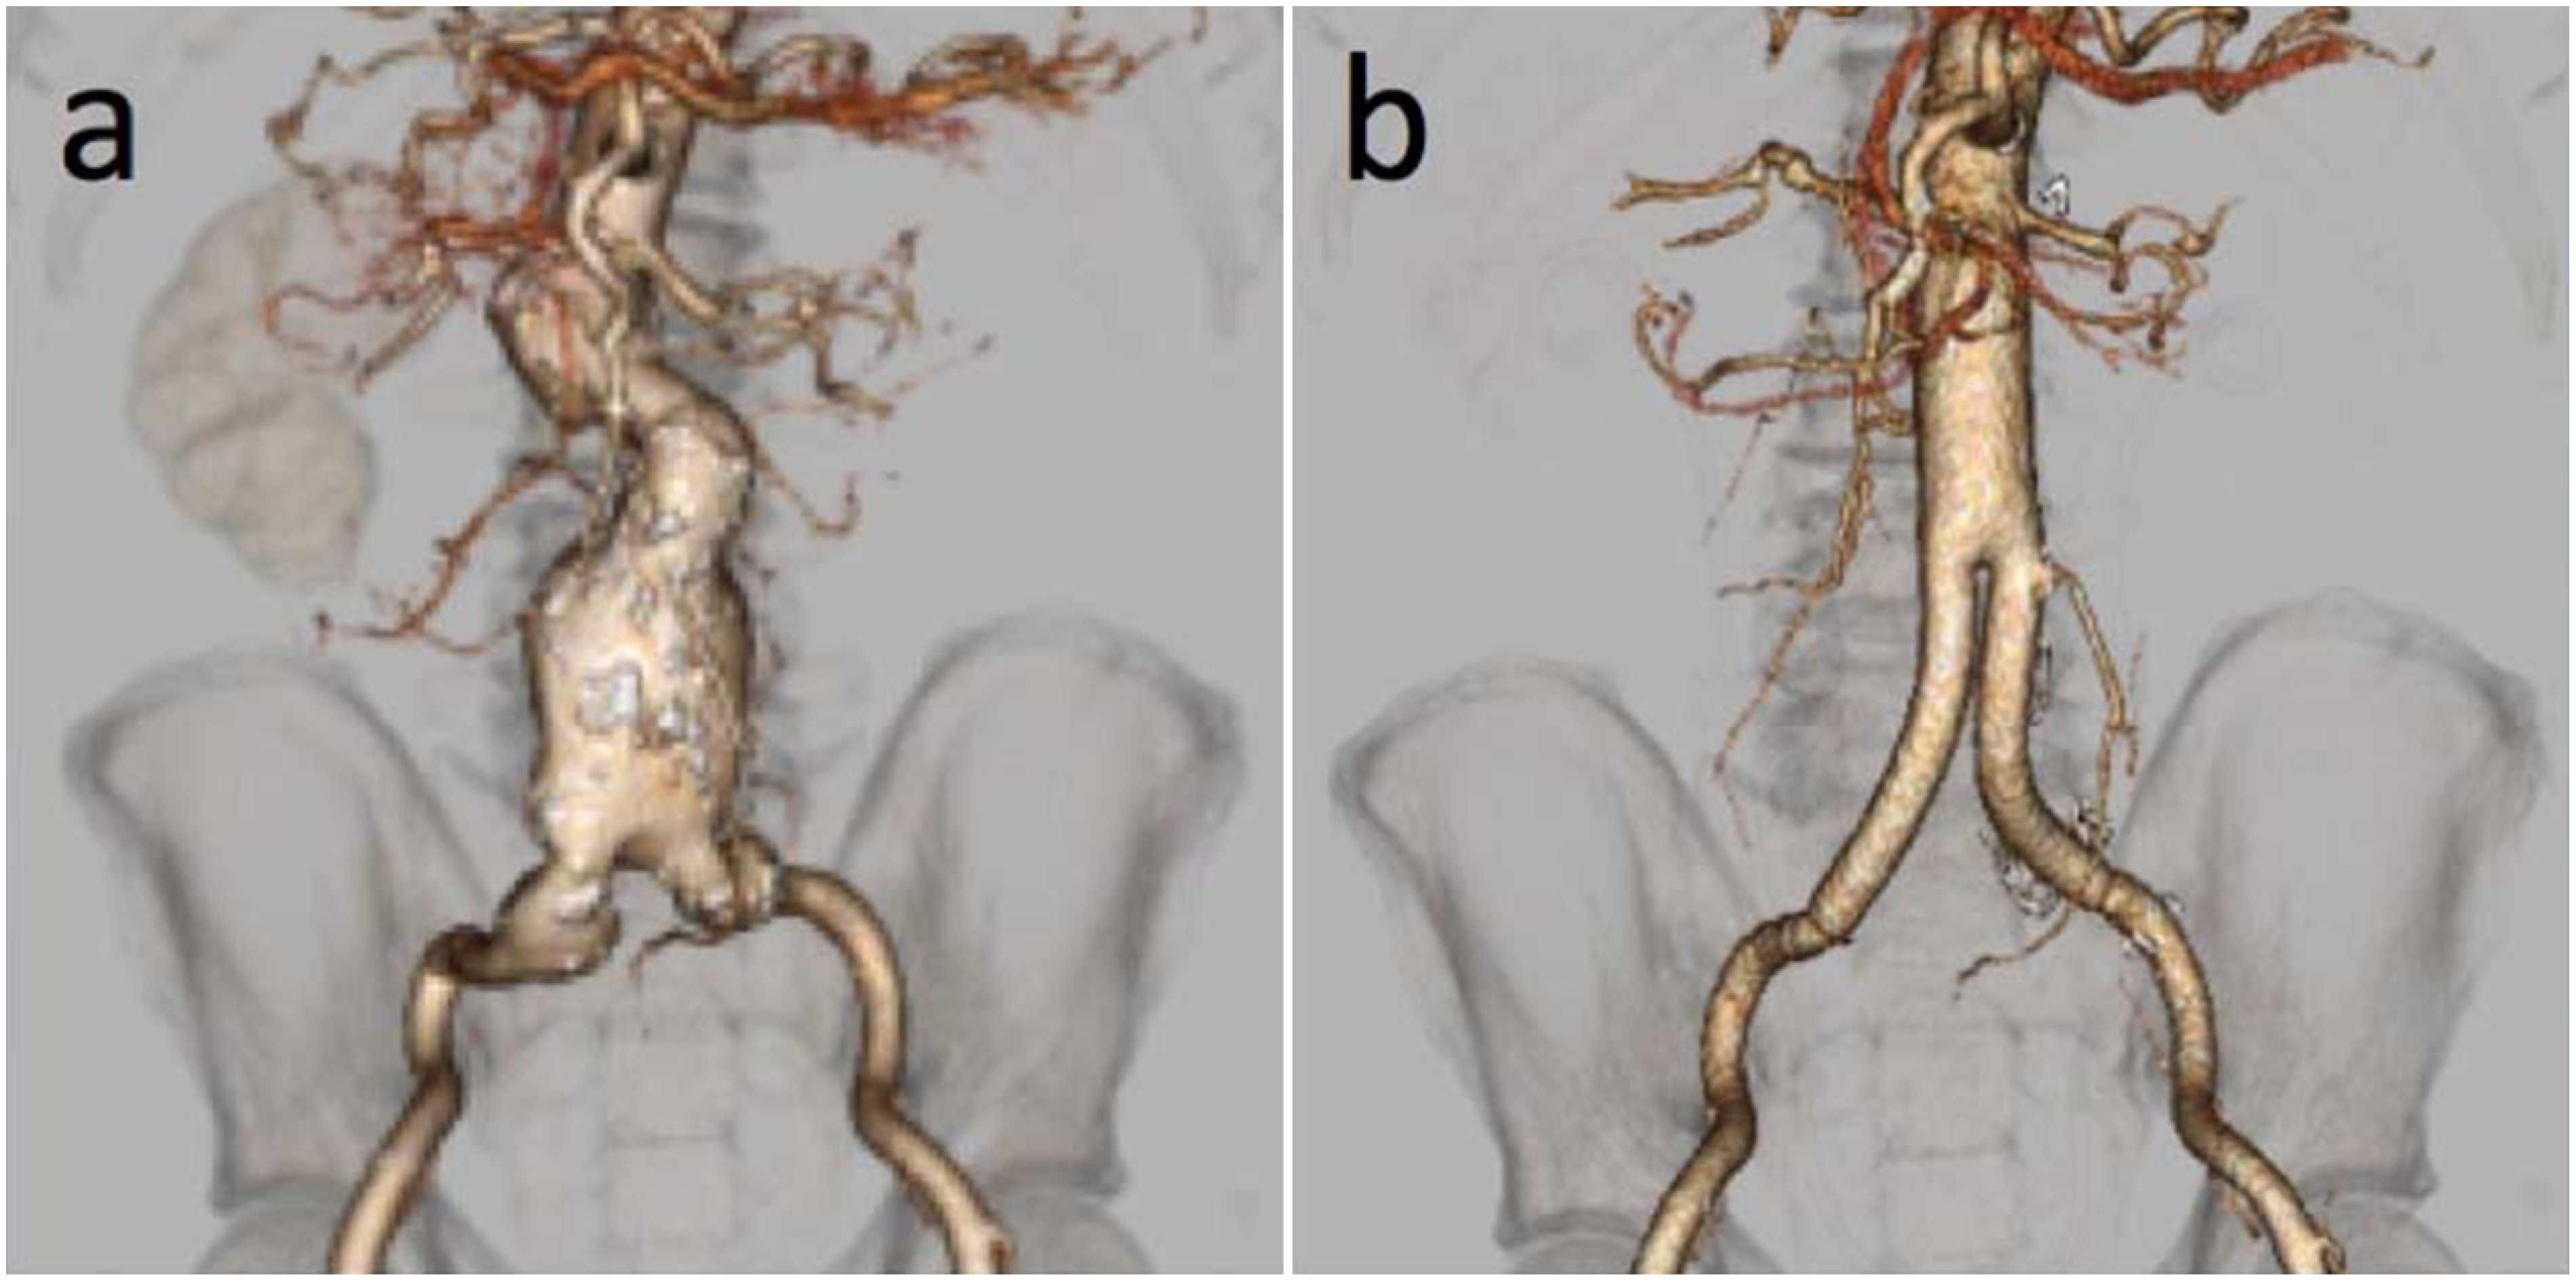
Fig. 3 (a) Preoperative 3-dimensional computed tomography (3DCT). (b) Postoperative 3DCT.
